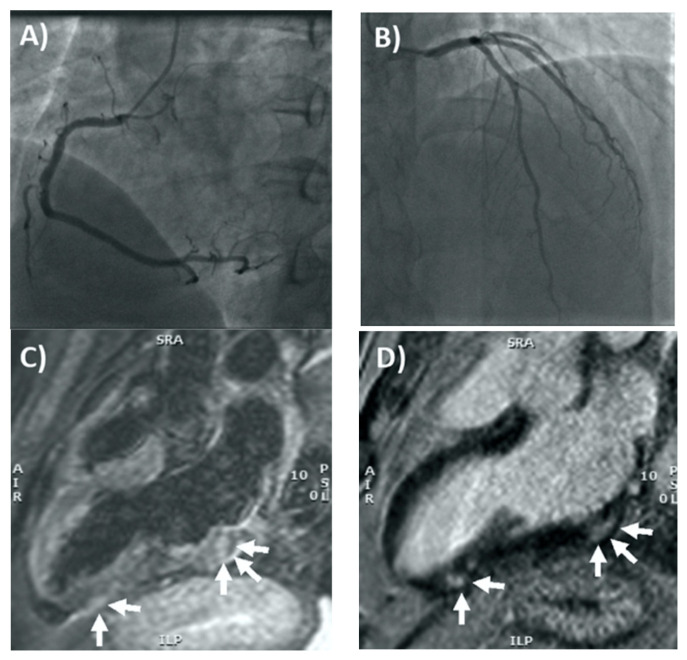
Coronary angiography and cardiac MRI. (A) The right coronary artery only had a mild plaque (<30% luminal diameter) in the mid portion, whereas in (B) the left main stem, left anterior descending artery, and circumflex artery had no evidence of coronary plaques. (C) T2-weighted 3-chamber view on cardiac MRI, showing focal areas of edema involving the subepicardial-intramyocardial regions of the basal and apical segments of the inferolateral wall (arrows). (D) Late gadolinium enhancement confirmed the presence of non-ischemic myocardial lesions in the basal and apical segments of the inferolateral wall (arrows) consistent with acute myocarditis. Reprinted/adapted with permission from Ref. [13] 2021, Elsevier.
